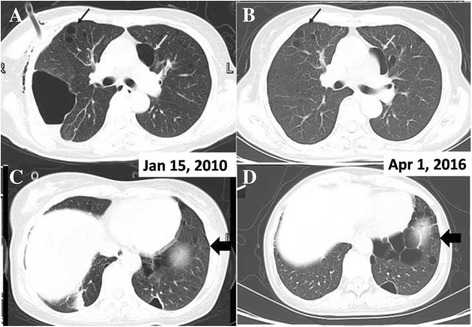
Postoperative changes are shown in the right lung. a, c The previous CT scan shows numerous cysts in the lung bases; one dominant cyst measures 8.4 cm × 5.1 cm in the right lung and contains some liquid; another huge cyst is anterior to the heart (*white arrow*). b, d The right lung expanded well after the operation with no obvious scar or atelectasis. Some cysts remained stable (*black arrow*) or became even smaller (*white arrow*). However, more cysts appeared in the lung bases (*thick arrow*)
